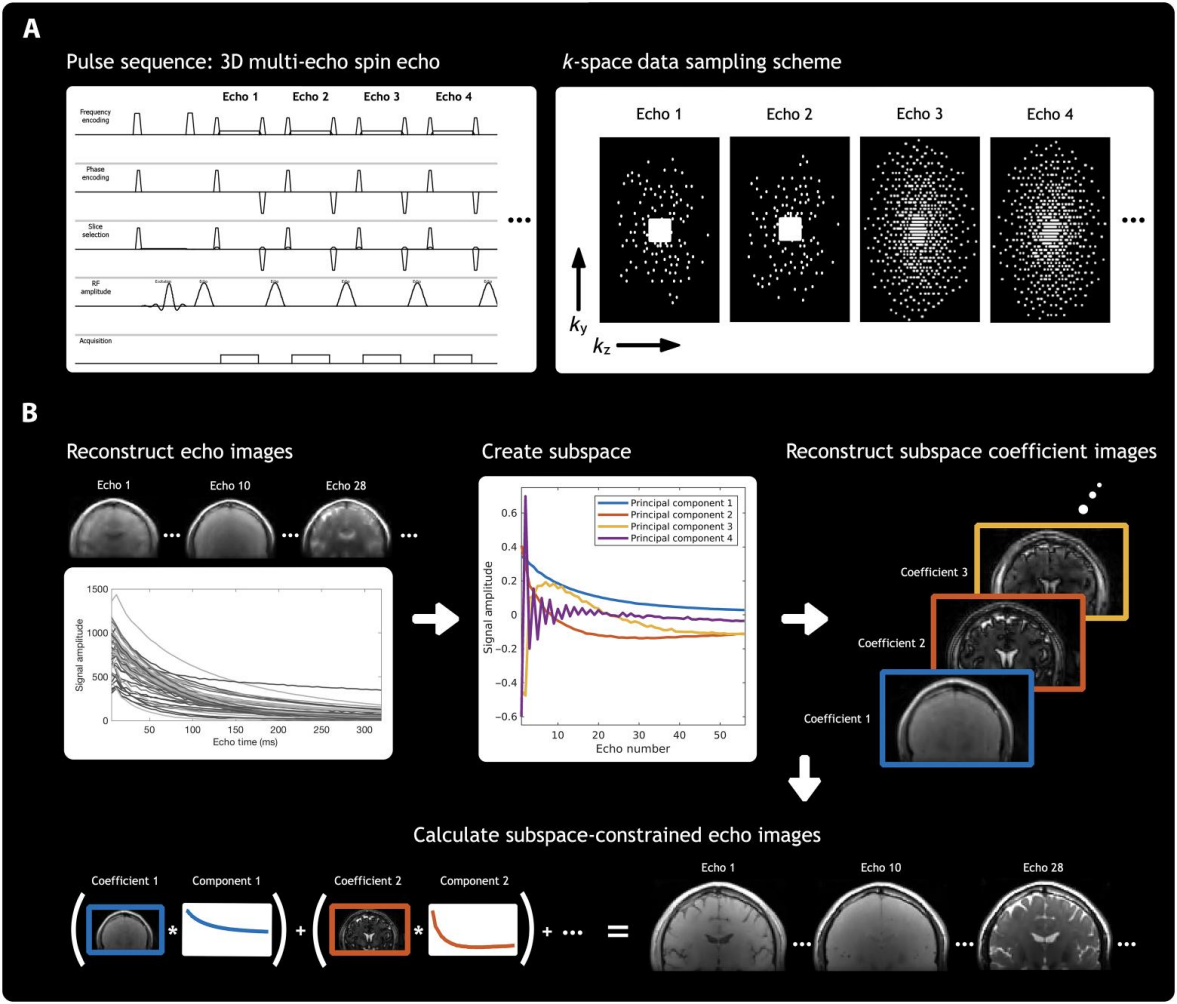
CALIPR framework acquisition and reconstruction. A graphical overview of the CALIPR framework methodology. For (A) acquisition and (B) reconstruction. For (A) image acquisition, the pulse sequence phase encoding gradients are modified to sample data incoherently across the additional nonspatial dimension present for many magnetic resonance imaging techniques. For (B) reconstruction, images from an initial naïve reconstruction are used to generate a subspace, calibrated for that specific dataset. A second and final reconstruction is then performed, explicitly constrained to this low-dimensional subspace. This combined acquisition and reconstruction framework exploits redundancy present in additional, nonspatial dimensions of the data by sampling in a way that makes under sampling artifacts less coherent and therefore more easily suppressed by a sparsity-promoting reconstruction, while true image features remain coherent and easily recoverable.
